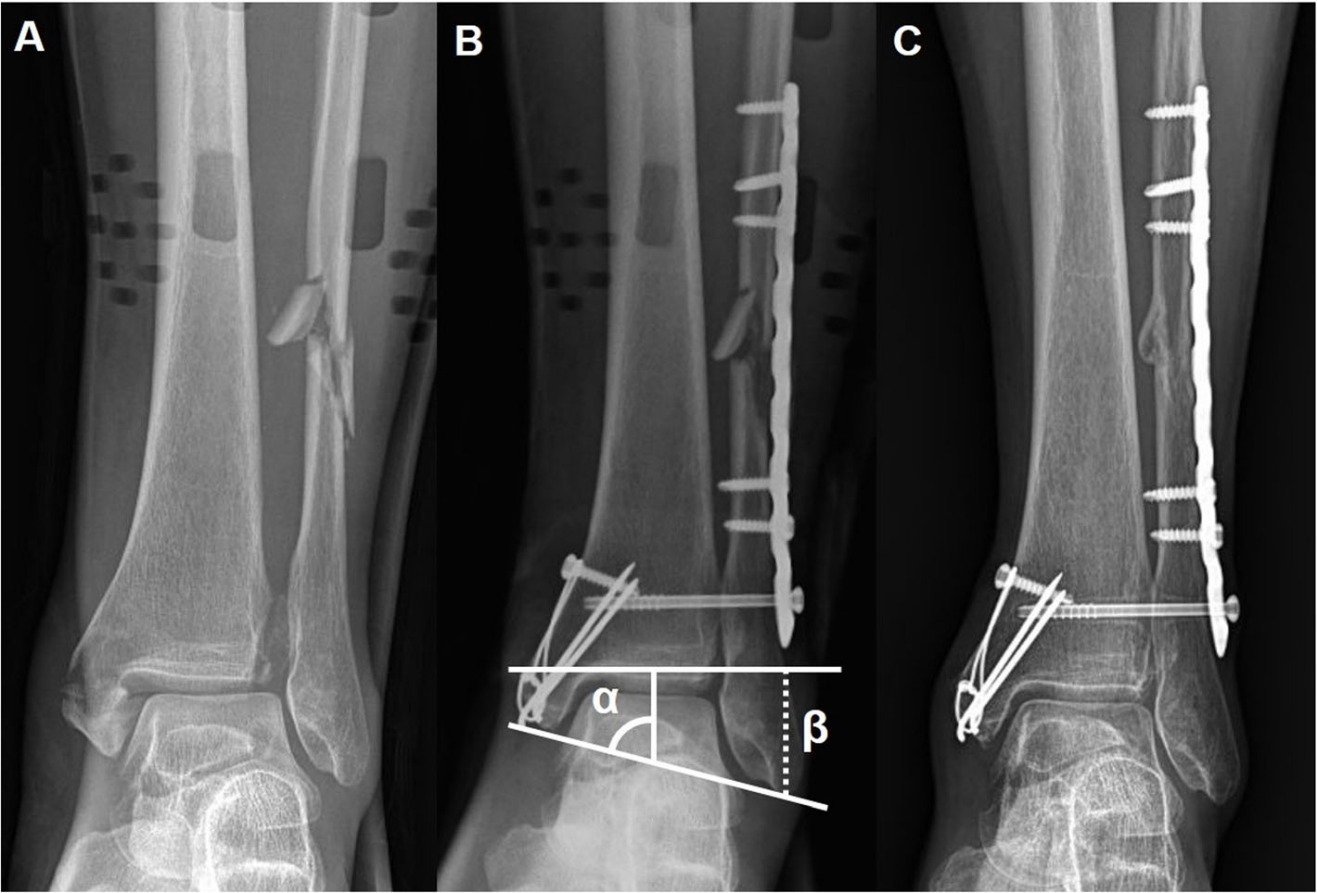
A preoperative radiograph shows a trimalleolar fracture with fibular comminution (a). A postoperative radiograph shows restoration of the length and radiologic parameters. Talocrural angle (*α*) was defined as the angle between the distal tibial articular line and the line connecting the tips of the distal fibula and medial malleolus. Fibular length (*β*) was defined as the distance from the distal fibular tip to the distal tibial articular line (b). One-year follow-up radiograph shows good union (c)
